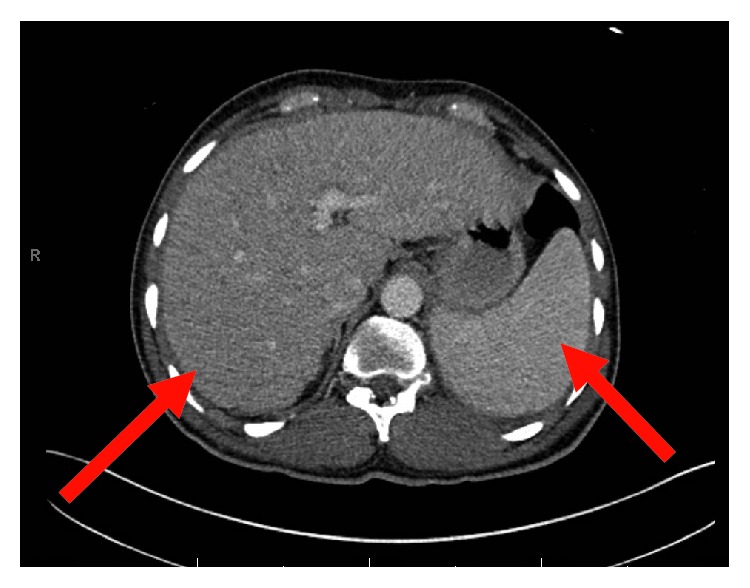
CT abdomen/pelvis showing hepatosplenomegaly.
